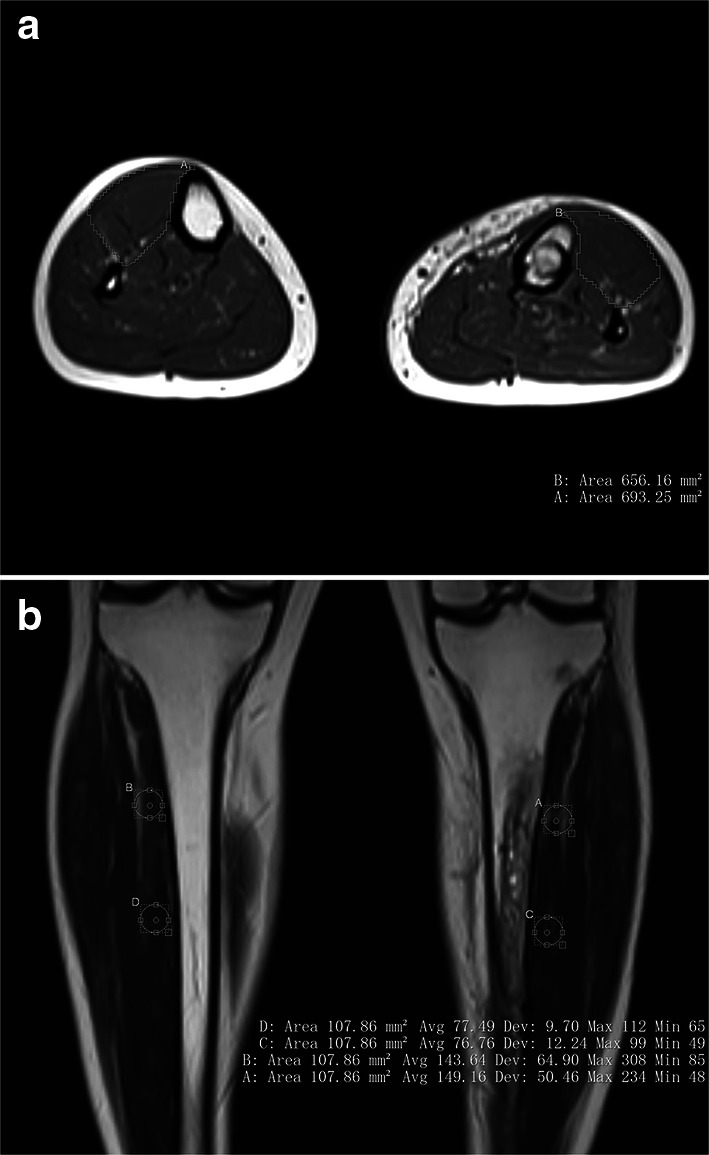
a The traced outlines of anterior compartments of the affected and normal limbs in the axial plane, which were used to measure anterior compartment cross-sectional areas (CSAs). The cross-sectional area ratio (CSAR) of the affected limb to the normal limb was calculated to evaluate the recovery of skeletal muscle volume. b T2-weighted image obtained in the coronal plane. Two regions of interest (ROIs), each with an area of 1 cm^2^, were chosen at identical locations in the affected and normal limbs . Vascular and fascial structures were kept outside the ROIs. The average T2-weighted signal intensity (T2SI) of the ROI was calculated for the affected limb and for the normal limb; the ratio of these T2SIs—the average T2-weighted signal intensity ratio (T2SIR)—was used to assess the recovery of skeletal muscle internal structures. Note the high-signal lesion in the medullary cavity of the affected tibia after the removal of the intramedullary nail
